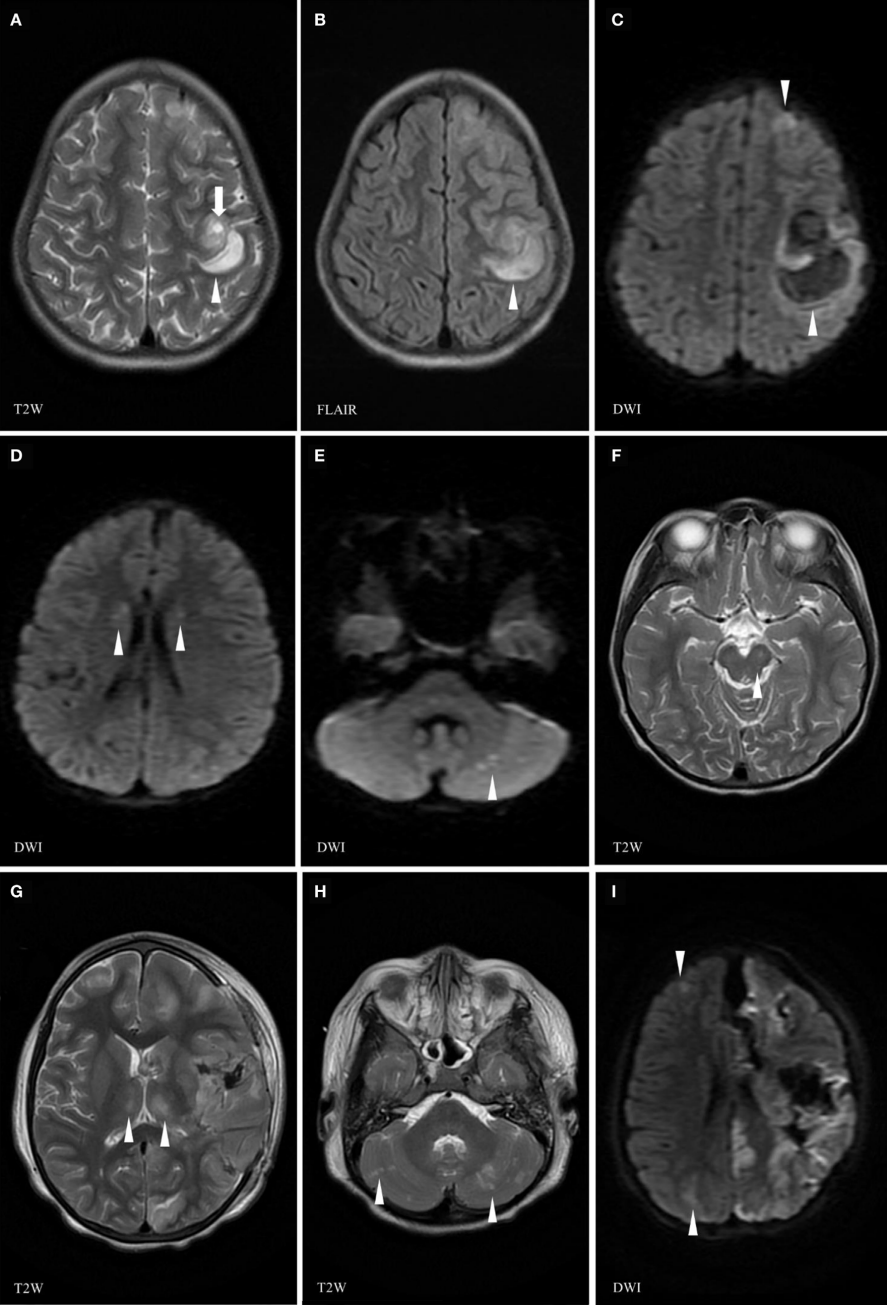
Brain magnetic resonance imaging (MRI) (day 30) at the time of the relapse of the neurological manifestations showing left frontoparietal cortical and subcortical areas of isointense T1, high T2 (A), FLAIR (B), and restricted diffusion (C) signals (arrowhead) increasing in size along the MRI examination, due to an active hyperacute bleed [arrow (A) pointing at spot sign indicating active bleed]. Area of high T2 and FLAIR signal changes (arrowheads) in the head of the caudate nucleus (D) and of abnormal diffusion weighed images (DWI) in the subcortical right cerebellar white matter (arrowheads) (E). Previous left midbrain lesion has resolved with only a tiny residual of high FLAIR signal corresponding to minimal residual gliosis (arrowhead) (F). Follow-up brain MRI at D33 showing a necrotico-hemorrhagic lesion in the left hemisphere (G,I), interval evolution of cortical/subcortical lesions, with new lesions in the bilateral thalami (G), cerebellar hemispheres (H), and right cerebral hemisphere (I) (arrowheads indicate abnormal signals).
